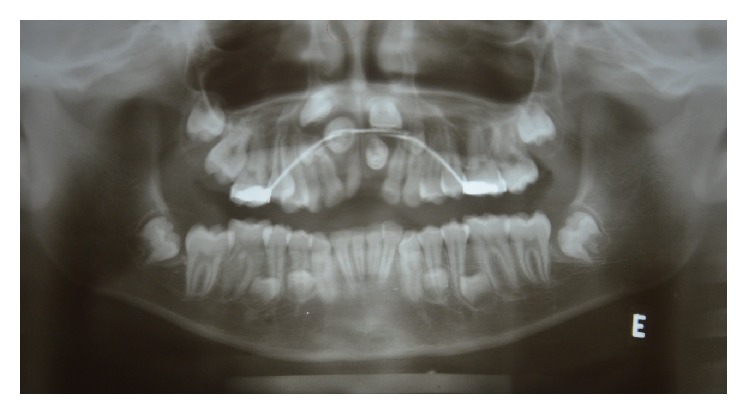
Orthopantomogram. Nine supernumerary teeth distributed in the maxillary and mandibular arches.
